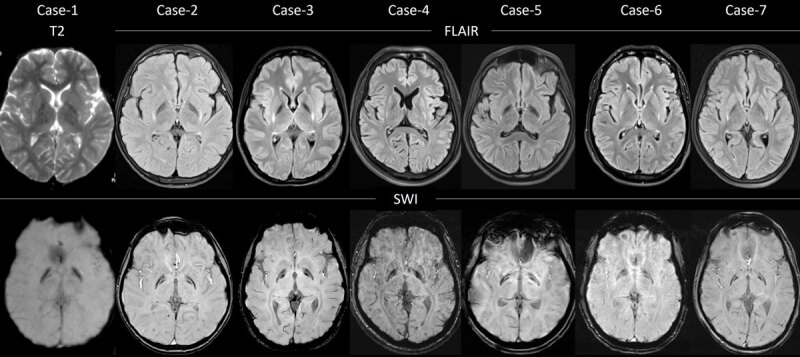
Magnetic resonance imaging of the brain. Axial T2, FLAIR (upper panel) and SWI sequences (Lower panel) of MRI brain of all the seven patients at the level of basal ganglia demonstrating bilateral symmetrical posterior putaminal atrophy and T2/FLAIR hyperintensity (upper panel) and mineralization of bilateral globus pallidi interna in the “wishbone” pattern on the SWI sequence (Lower panel). FLAIR: Fluid attenuated inversion recovery; MRI: Magnetic resonance imaging; SWI: susceptibility weighted imaging.
